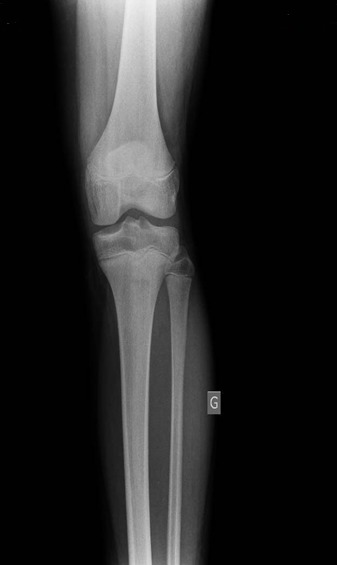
Radiographie de face du genou gauche
